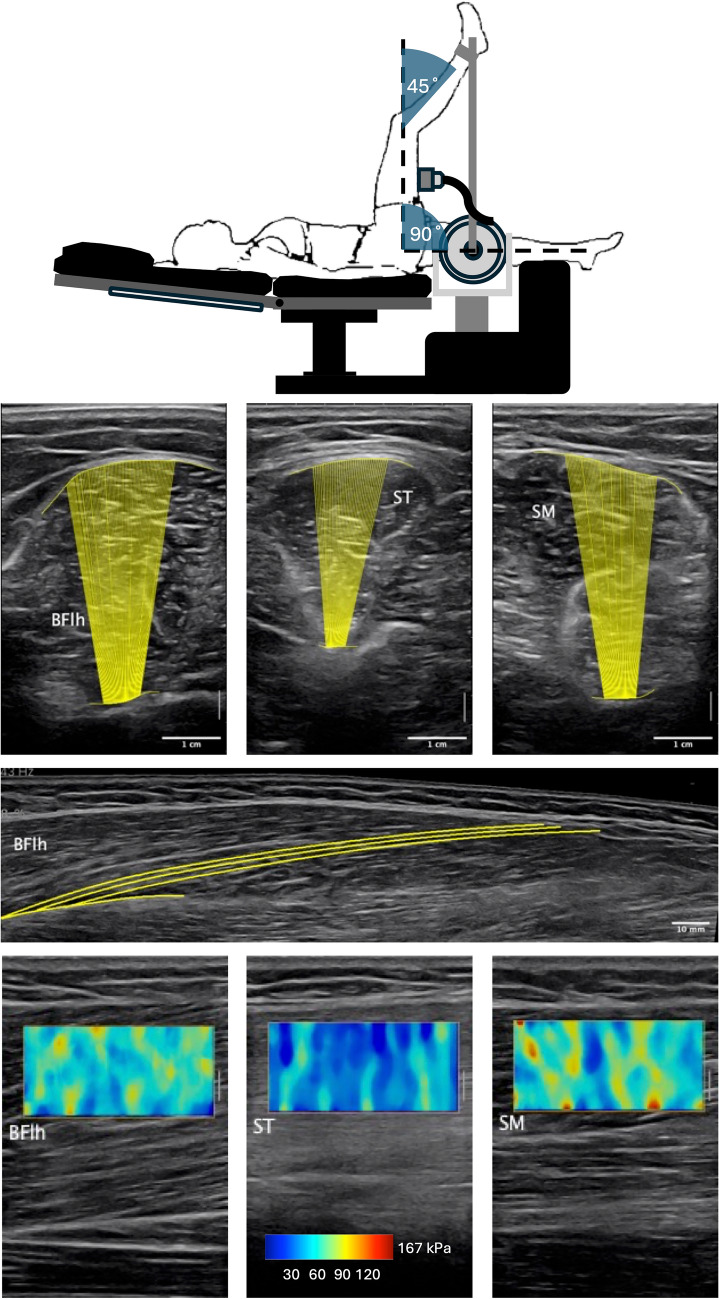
Experimental setup and representative ultrasound scans collected for the measurement of muscle thickness (1st row), fascicle length (2nd row) and muscle shear modulus (3rd row). BFlh: biceps femoris long head muscle, ST: semitendinosus muscle, SM: semimembranosus muscle
